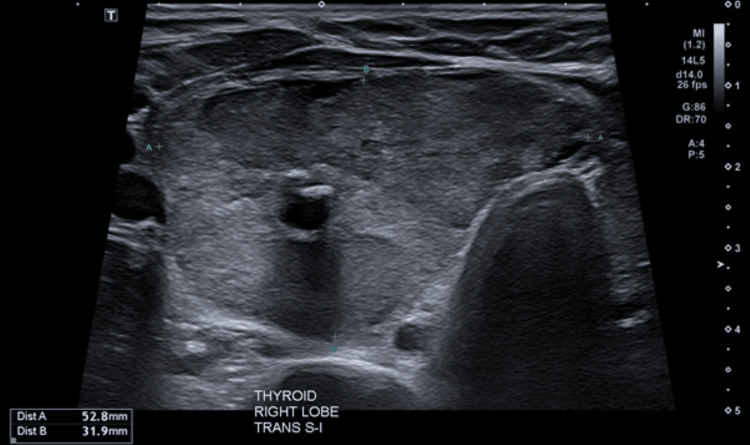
Ultrasound of the neck showing a dominant right-sided thyroid lesion measuring 62 x 52 x 32 mm.
